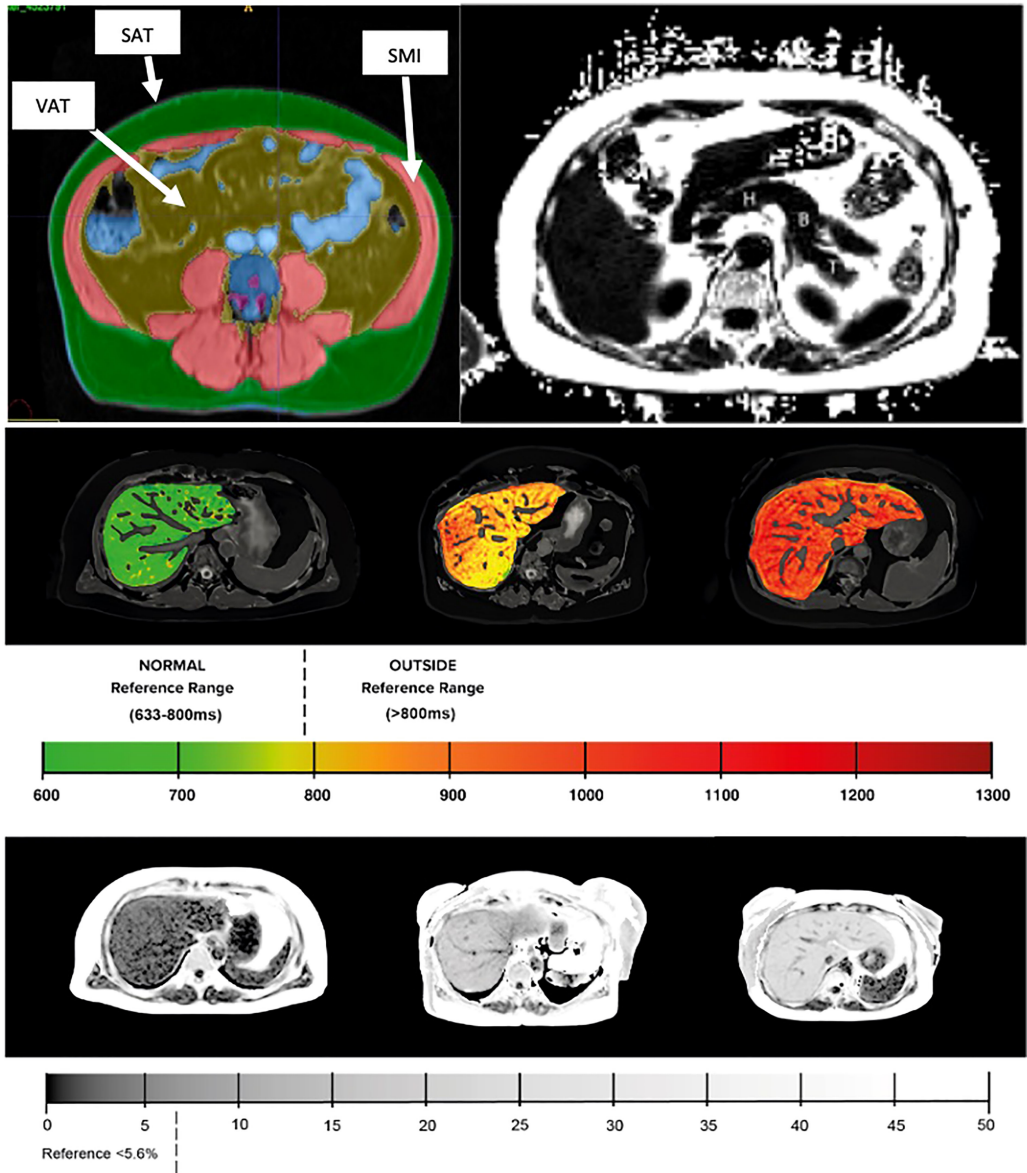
(Top left) Example MR images of body composition segmentation: SMI, skeletal muscle index; SAT, subcutaneous adipose tissue; VAT, visceral adipose tissue. (Top right) pancreas with example typical ROI placement (H – head, B – body, T – tail). (Middle) liver cT1 and (bottom) liver PDFF with corresponding reference values.
